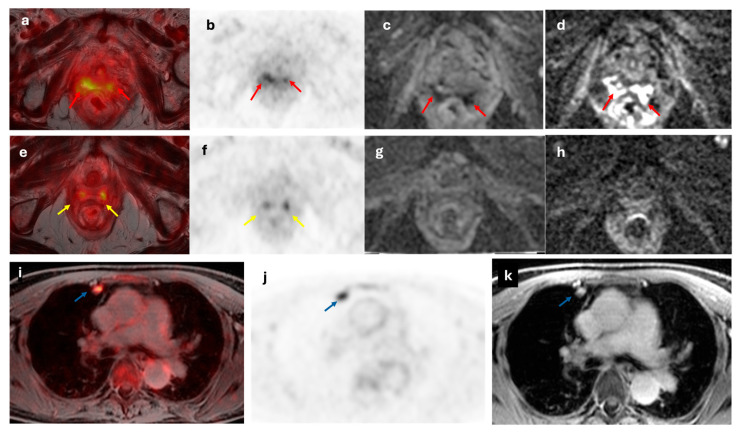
A 64-year-old woman with recurrent EC at vaginal stump (red arrow). The lesion demonstrated increased metabolic activity on axial fused [^18^F]F-FDG PET/MRI and axial [^18^F]F-FDG PET images (a,b), along with diffusion restriction on axial ADC and DWI images (c,d). The lateral vaginal walls show diffuse increased metabolic activity (yellow arrows; (e,f)) with no diffusion restriction (g,h) consistent with inflammatory changes secondary to radiotherapy. Axial fused [^18^F]F-FDG PET/MRI, axial [^18^F]F-FDG PET and axial T2-weighted MRI images (i–k, respectively) show a hypermetabolic nodule in the right upper lung lobe (blue arrow), suggestive of malignancy.
